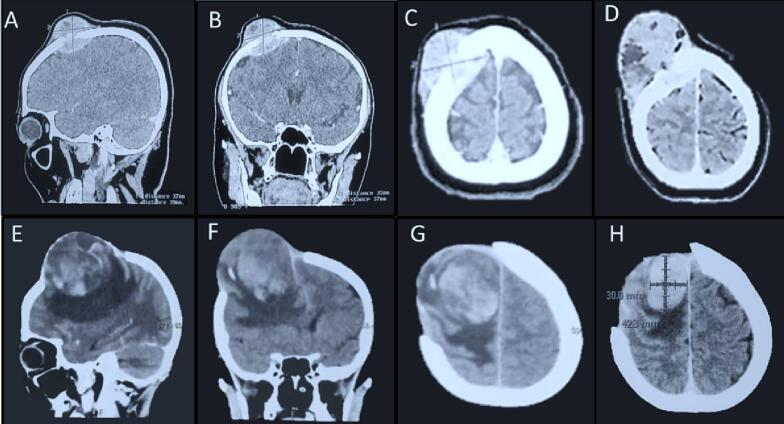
A–C. First head CT with contrast, there was a solid mass, D. Evaluation of head CT after two months, E-F. Head CT examination one month after surgical resection, H. The progression of head CT evaluation after one week.
